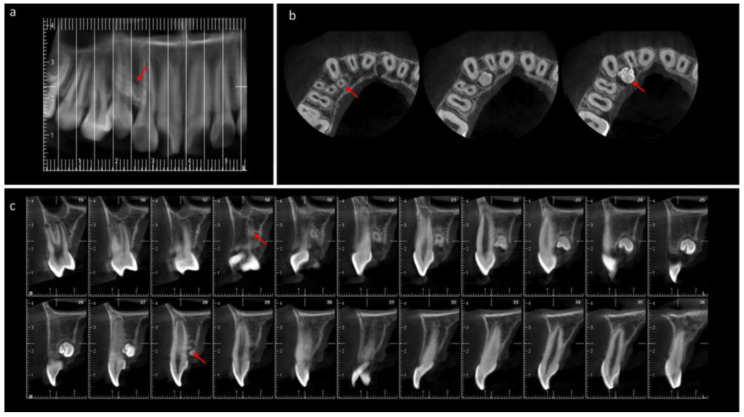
Cone-beam computed tomography (CBCT) of the maxilla. 2021, five years after the first surgery. (a) Panorex section. Presence of a radiopaque area, compatible with odontoma, overprojected to teeth 12 and 13, mesioangular. (b) Axial section. Multilobulated odontoma, located palatal to teeth 12 and 13, surrounded by a radiolucent demarcation line. Discrete external resorption in the cervical third of the palatal root of tooth 13. (c) Cross-section. Presence of odontoma (Sections 18–28) in intraosseous evolution, microdontic, mesioangular, coronal structural alteration. Palatally displaced tooth location with thinning and perforation of palatal bone cortex. The crown is located palatally and in contact with the cervical third palatal root of tooth 12 and the cervical and middle third palatal root of tooth 13. Root location is palatally displaced. Root middle third in contact with palatal root middle third of tooth 13. Pericoronary sac and periodontal ligament space of preserved thickness (scale 100%). Red arrow = odontoma.
